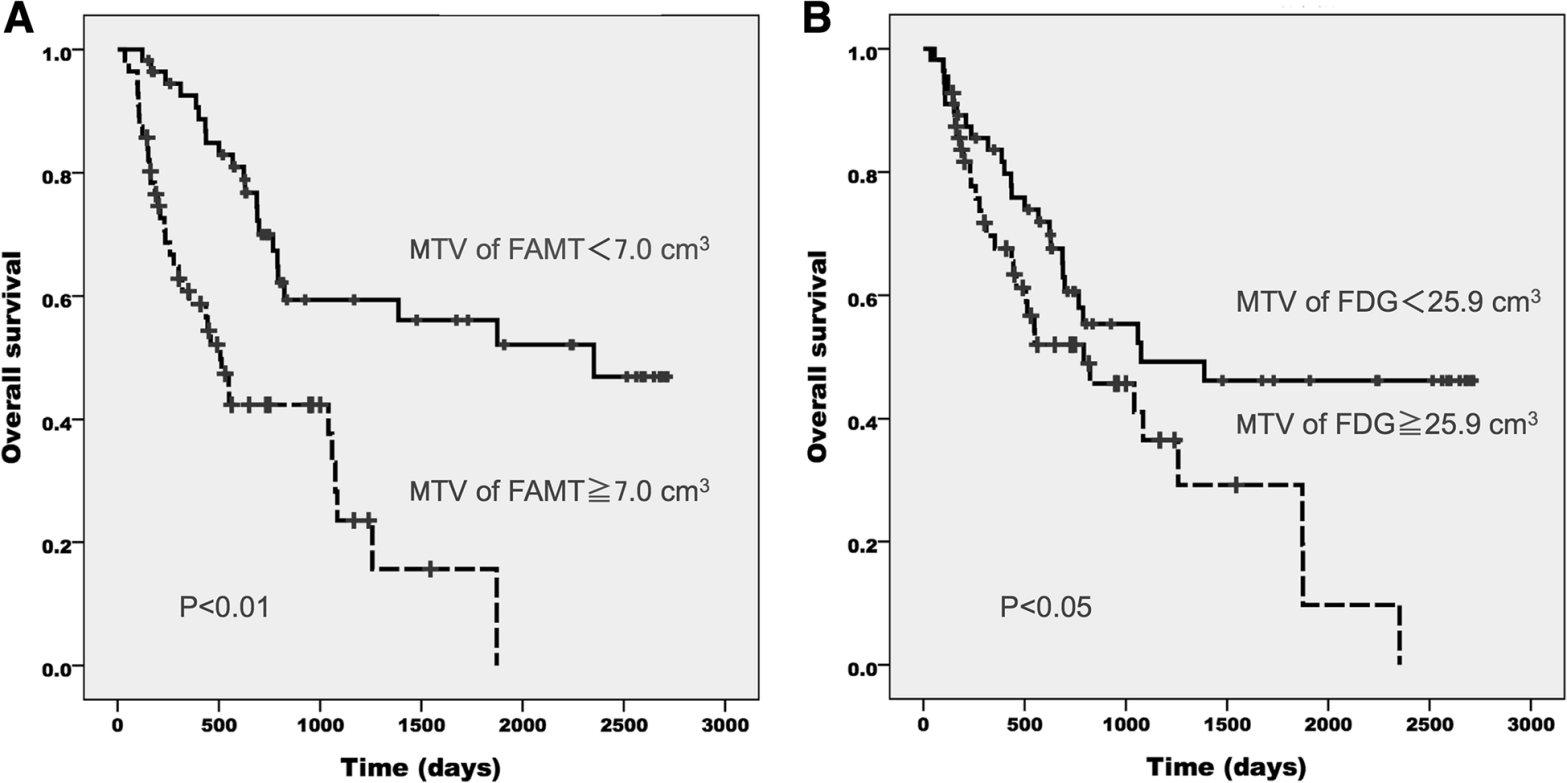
Overall survival of NSCLC patients according to the MTV of ^18^F-FAMT (a) and ^18^F-FDG (b)
